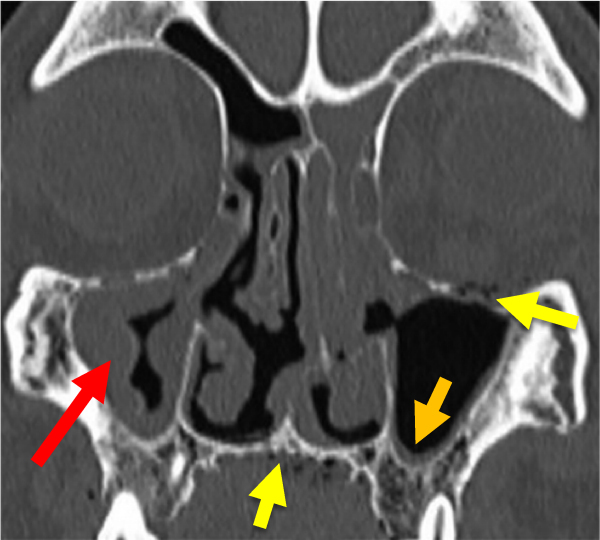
Computed Tomography of the Paranasal Sinuses (Coronal Plane, Bone Window). Polyps (red arrow) and mucosal thickening (orange arrow) can be seen in the right maxillar sinus and left ethmoid sinus. Intra- and extraosseous air bubbles (yellow arrows) indicate bone invasion.
